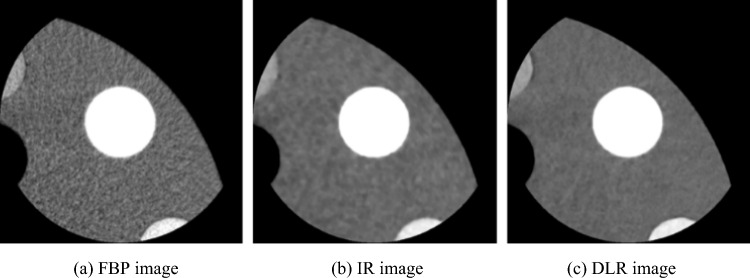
CT images of iodine-equivalent module reconstructed using three noise reduction algorithms (Window level: 40 HU, Window width: 400 HU)
